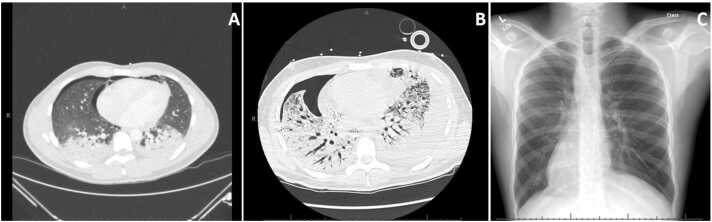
Chest CT (lung window). (A) Initial presentation after 1 month of Bactrim therapy; (B) on transfer after 2 months of Bactrim therapy; (C) post-transplant chest X-ray. CT, computed tomography.
